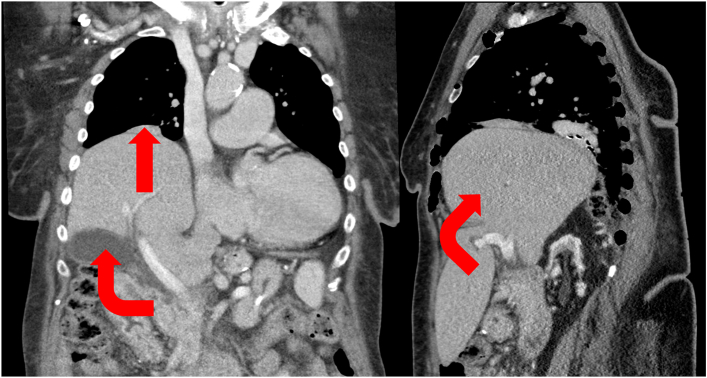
Preoperative portal-venous phase computed tomography (coronal and sagittal reformations) demonstrated the congenital diaphragmatic hernia with herniation of the liver into the thoracic cavity up to the level of the pulmonary hilum (straight arrow). The liver was rotated by approximately 90° (curved arrows), which raised concern for potential compromise of the hepatic hilum during intraoperative reduction.
